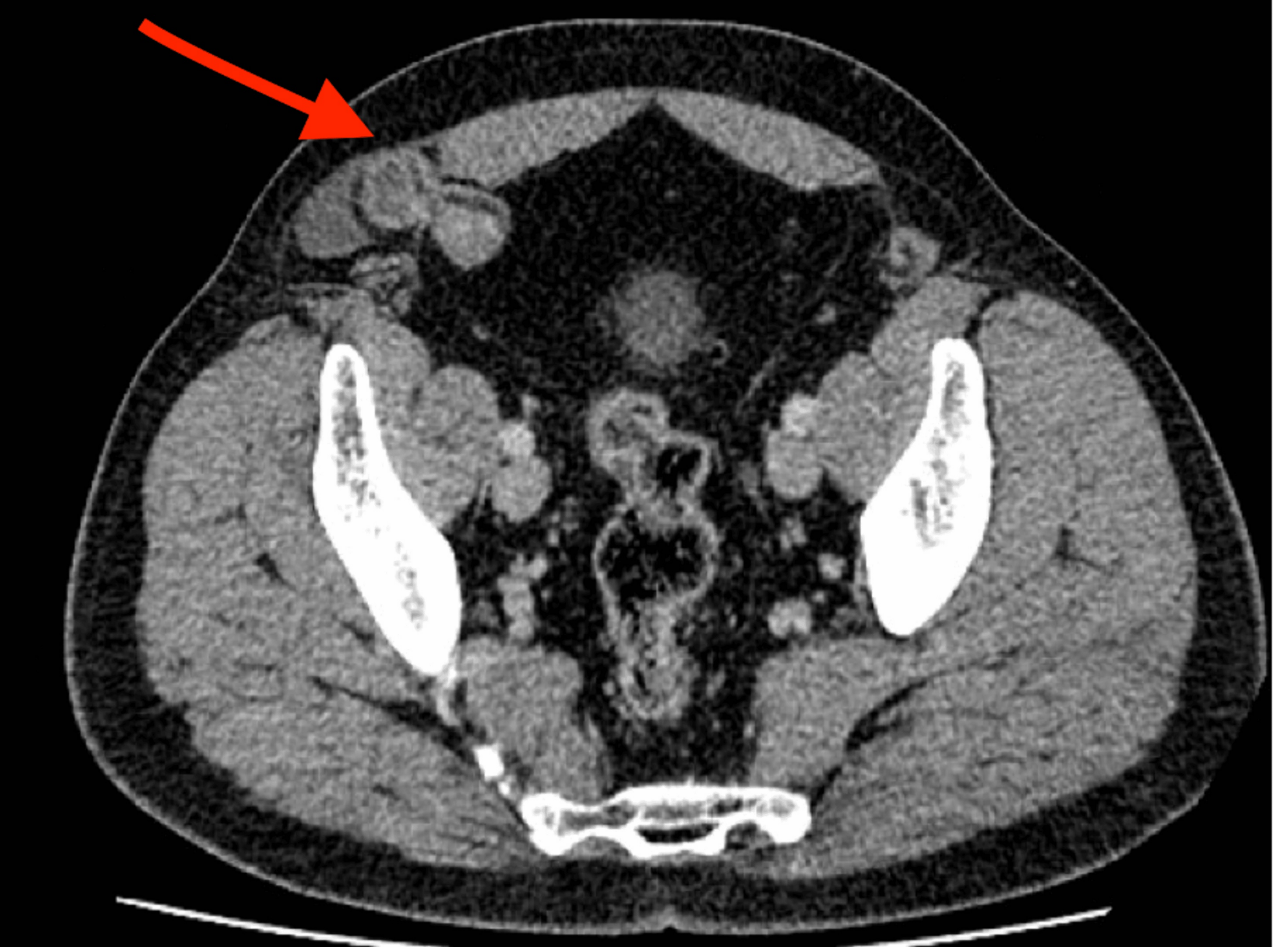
Axial contrast-enhanced CT of the abdomen demonstrates a right-sided abdominal wall defect through the Spigelian fascia, lateral to the rectus abdominis muscle (red arrow), containing a loop of small bowel. This finding is consistent with a right-sided Spigelian hernia.
